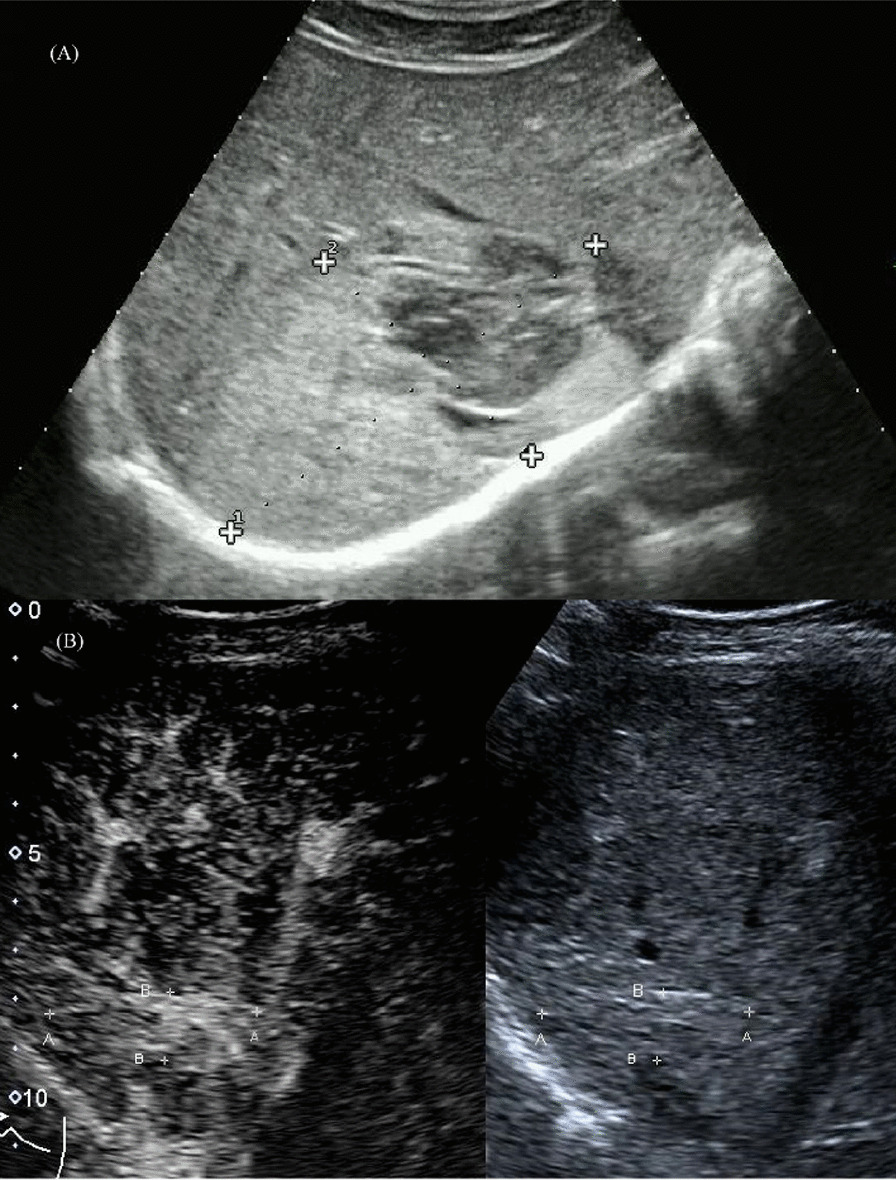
A 33-year-old male with a giant hepatic hemangioma of 10.0 cm in diameter. A Preoperative ultrasound (size: 10.0 cm × 6.1 cm). B Ultrasound after 12 months of the second percutaneous sclerotherapy. (size: 4.3 cm × 1.4 cm)
